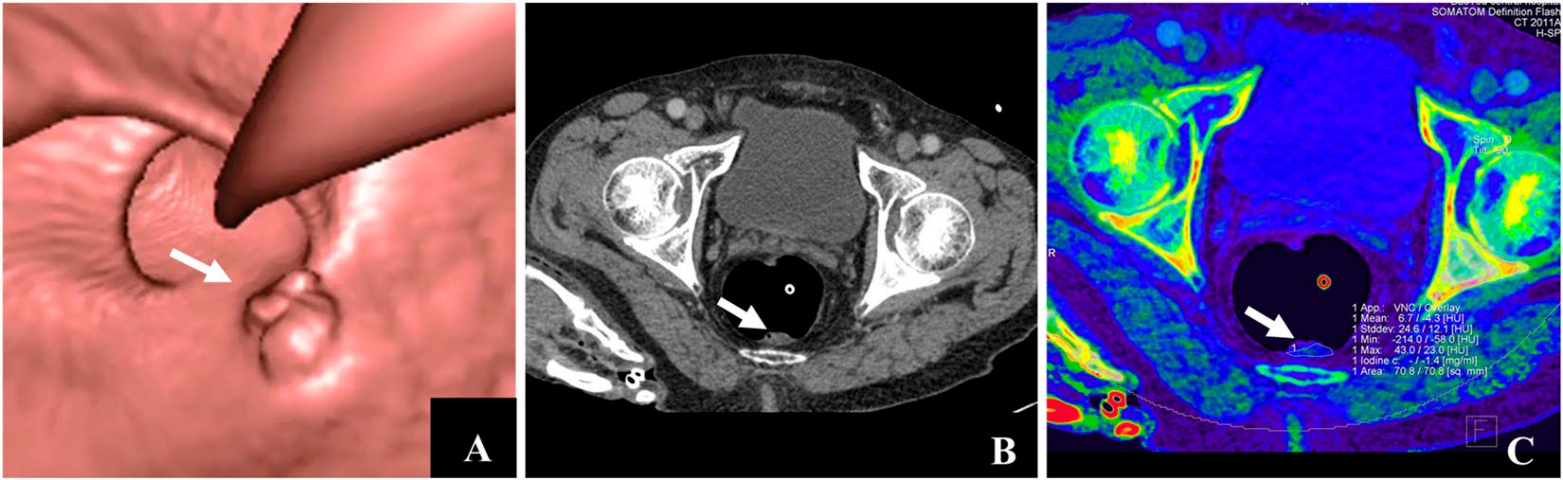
Differentiation from stool and colonic neoplasia by dual-energy CT. Male, 88 years of age. (A,B) denote colonography and CT axial plane image revealed irregular upheaval masses in the posterior wall of the rectum (white arrow). It is difficult to differentiate tumors from stool. (C) Dual-energy contrast-enhanced CT (axial plane) revealed no enhancement of masses, iodine value = −1.4 mg/ml (white arrow), suggesting no blood supply to the masses, which was validated as stool by colonography.
